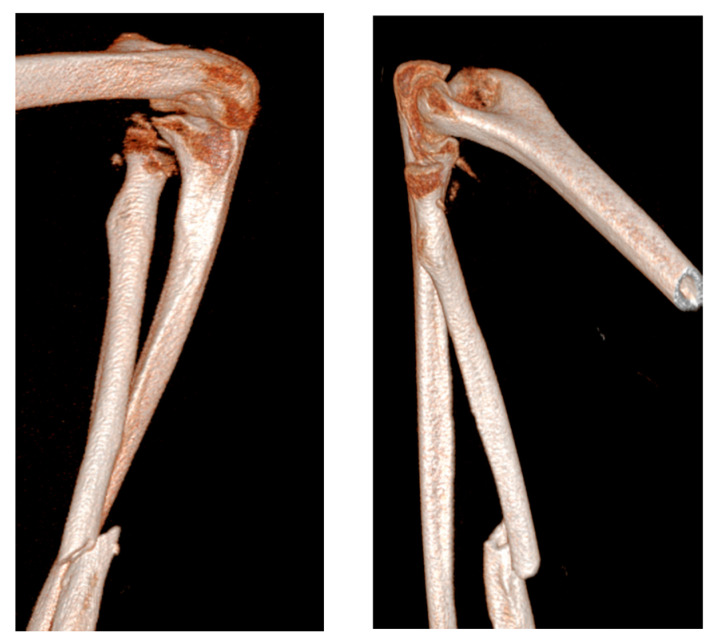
CT-based 3D model performed at the emergency room entrance.
